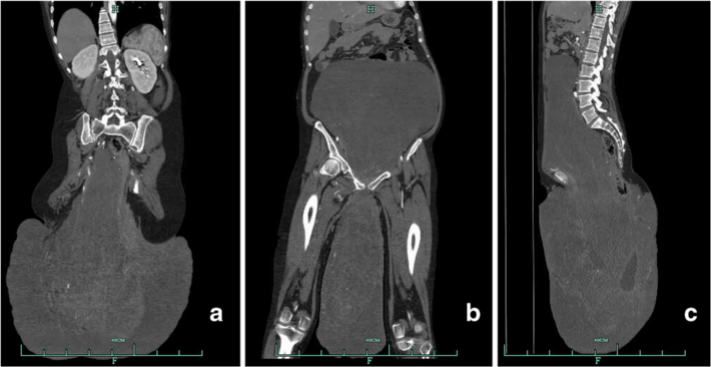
a–c CT revealed an enormous dumbbell-shaped mass (68.3 cm superoinferiorly) occupying the mid-abdomen
